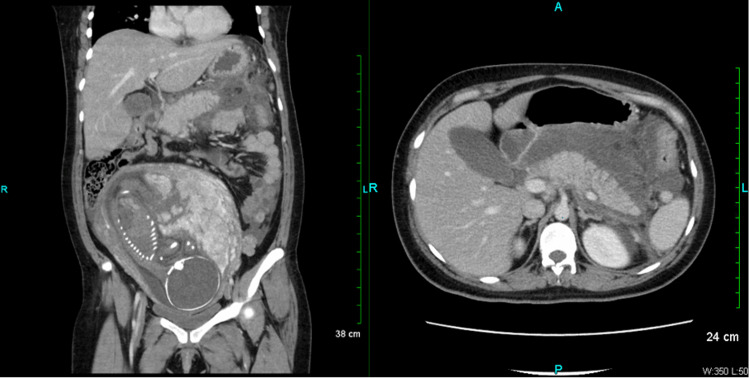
Coronal section (left) and axial section (right). Computed tomography scan of the abdomen and pelvis with contrast showing acute interstitial edematous pancreatitis with extensive peripancreatic fluid extending throughout the upper abdomen. No evidence of pancreatic necrosis.
